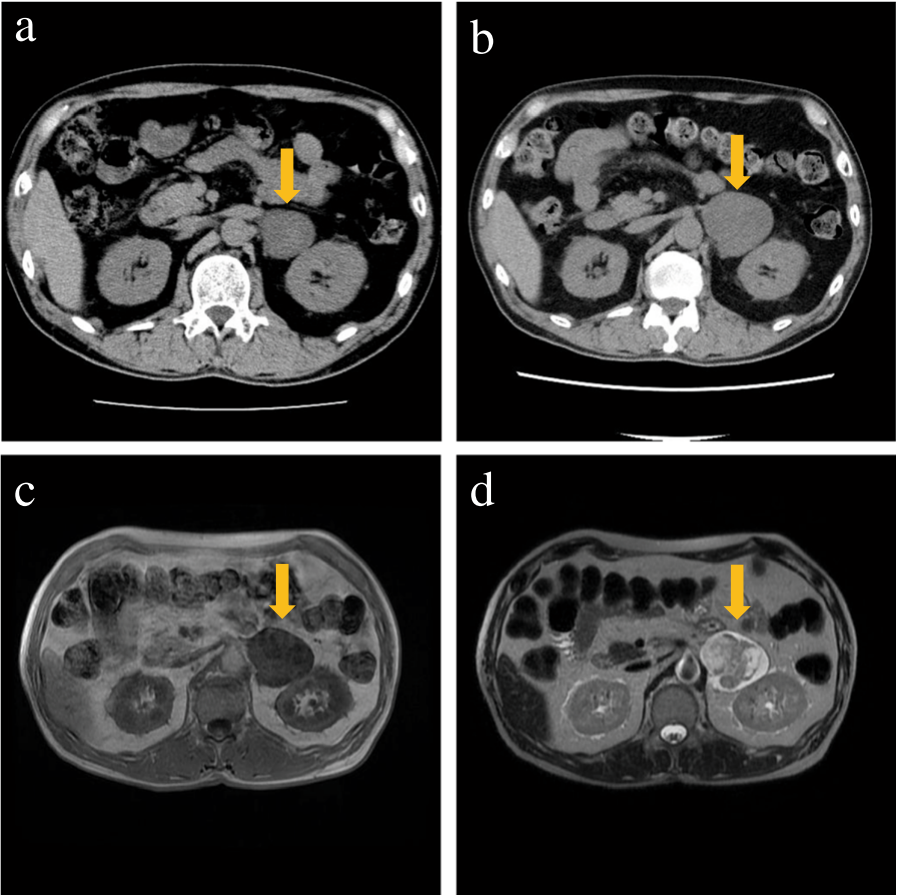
CT of the left adrenal area showing a mass (4.5 × 3.8 cm) when the patient was 60 years old (a) and a larger mass (5.8 × 5.2 cm) when he was 69 years old (b). MRI of the left adrenal area on T1-WI (c) and T2-WI (d) when the patient was 69 years old. Yellow arrows show the lesions of the adrenal area
